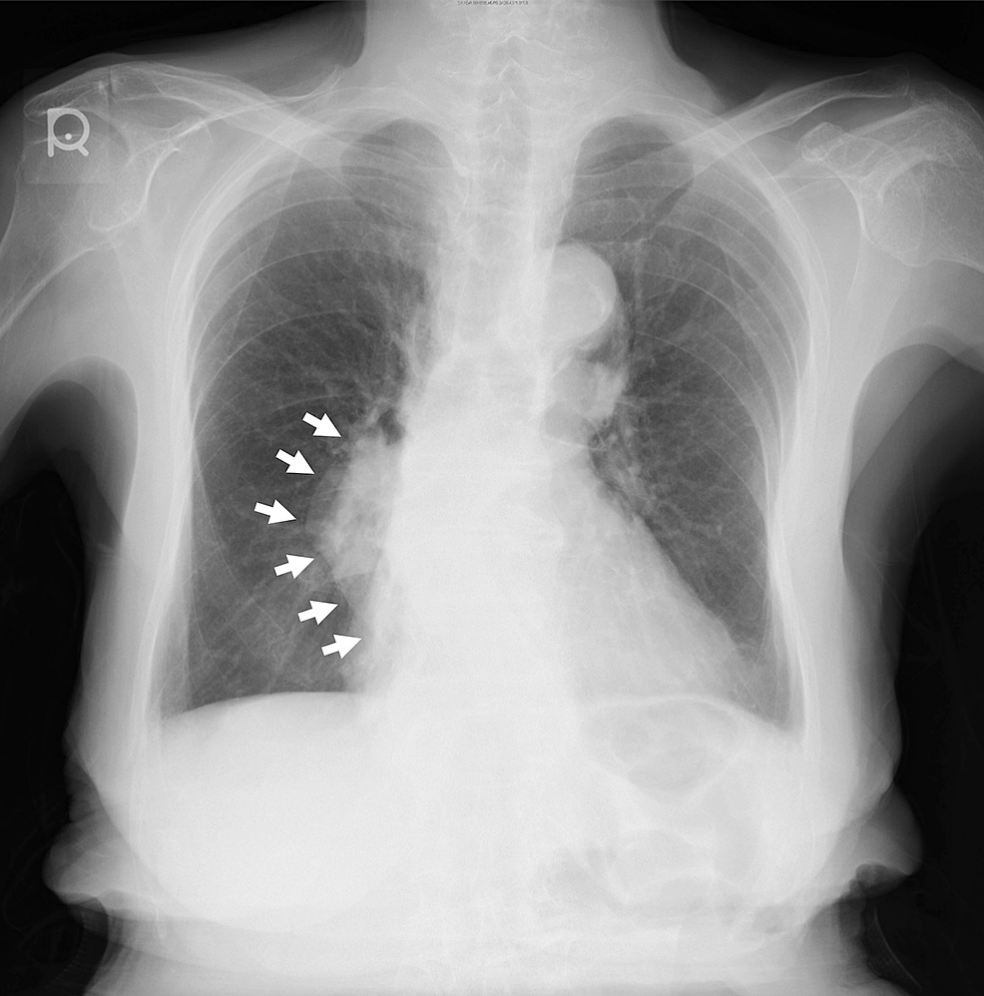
Chest radiography demonstrating abnormal shadowing and mediastinal enlargement.
